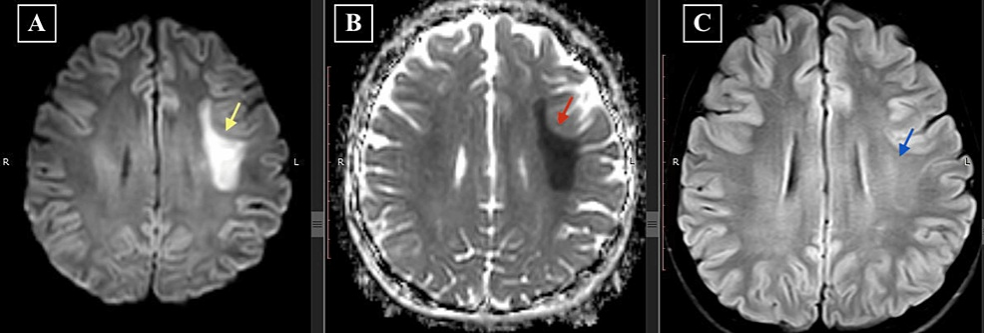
MRI brain on the day of onset of symptoms (A) Diffusion-weighted MRI image showing an area of restricted diffusion in the left frontal centrum semiovale and corona radiata (yellow arrow). (B) The area of restricted diffusion is hypointense on the ADC map (red arrow). (C) FLAIR image showing minimal hyperintensity in the same region (blue arrow). MRI, magnetic resonance imaging
